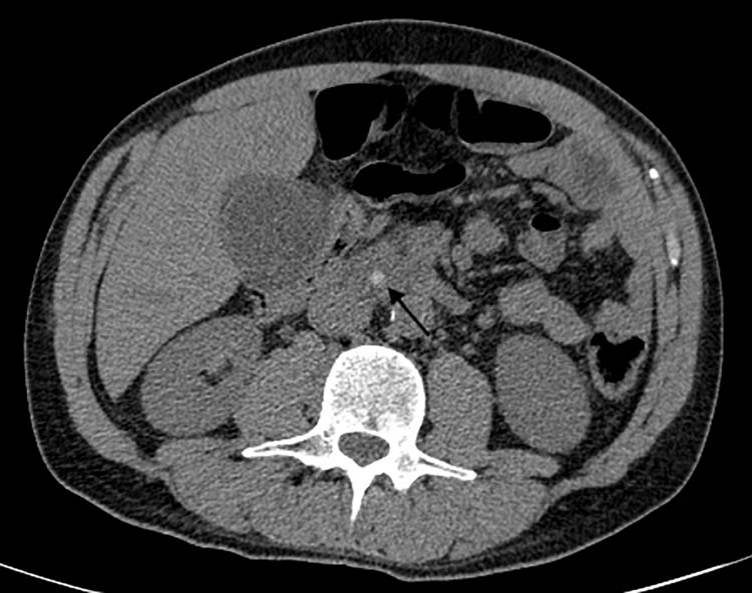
Computed tomography scan image showing stone in CBD.
